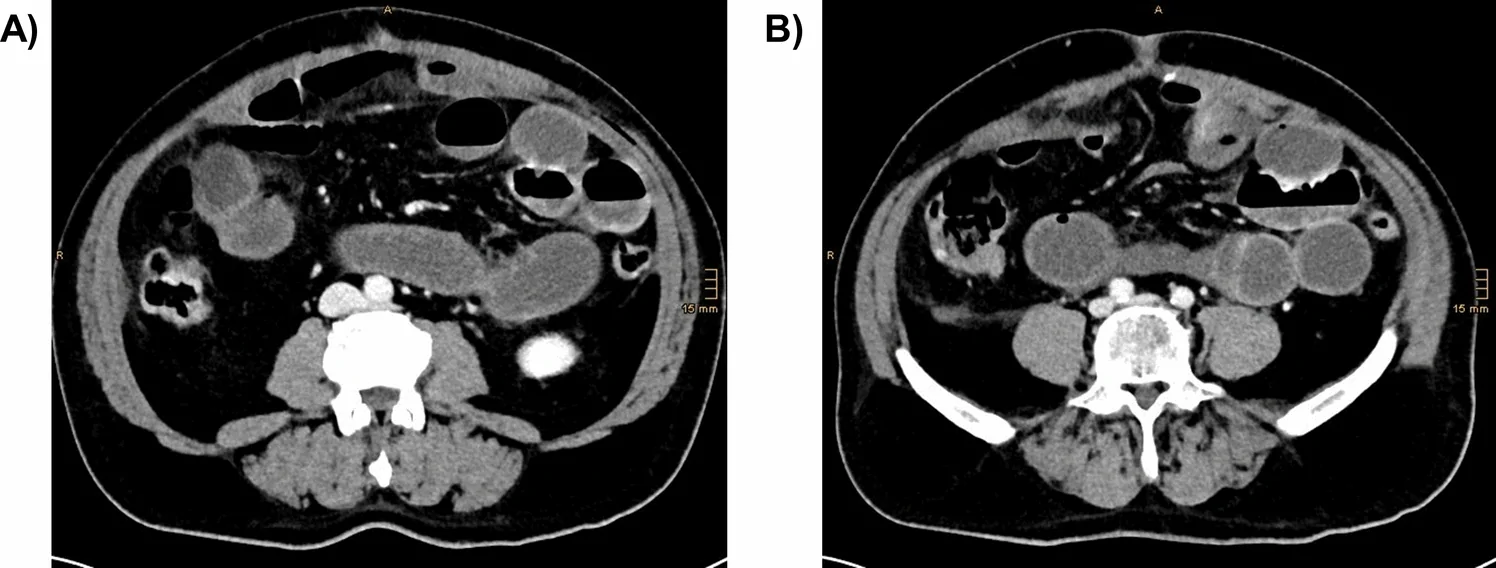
A and B Example CT scan of an interparietal hernia due to a posterior sheath dehiscence after eTEP Rives–Stoppa repair. Emergency CT scan upon suspicion is indicated with immediate surgical revision (usually laparoscopic management will be possible) due to possible incarceration of bowel
